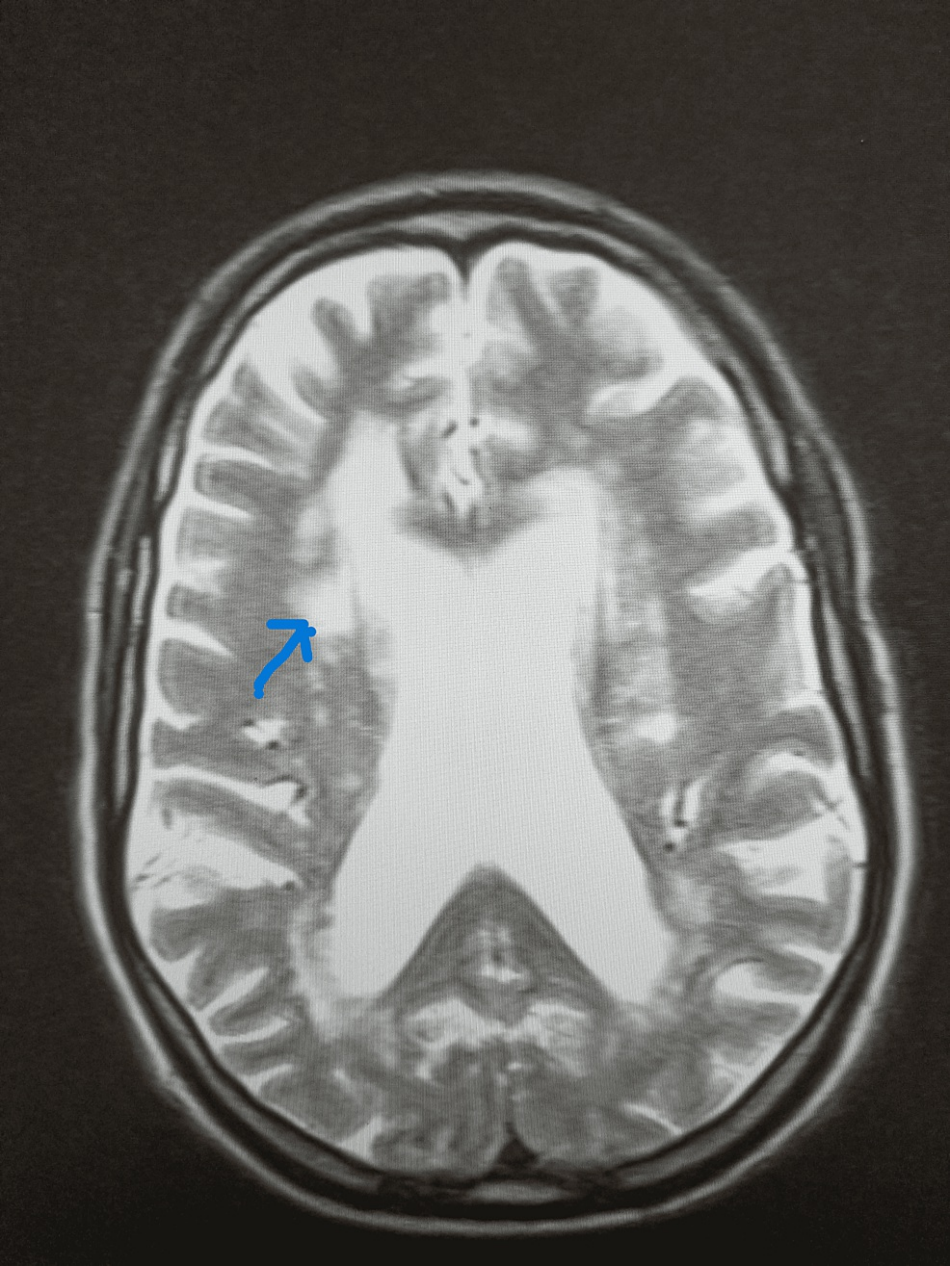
MRI showing nonspecific periventricular and deep white matter degenerative changes.
